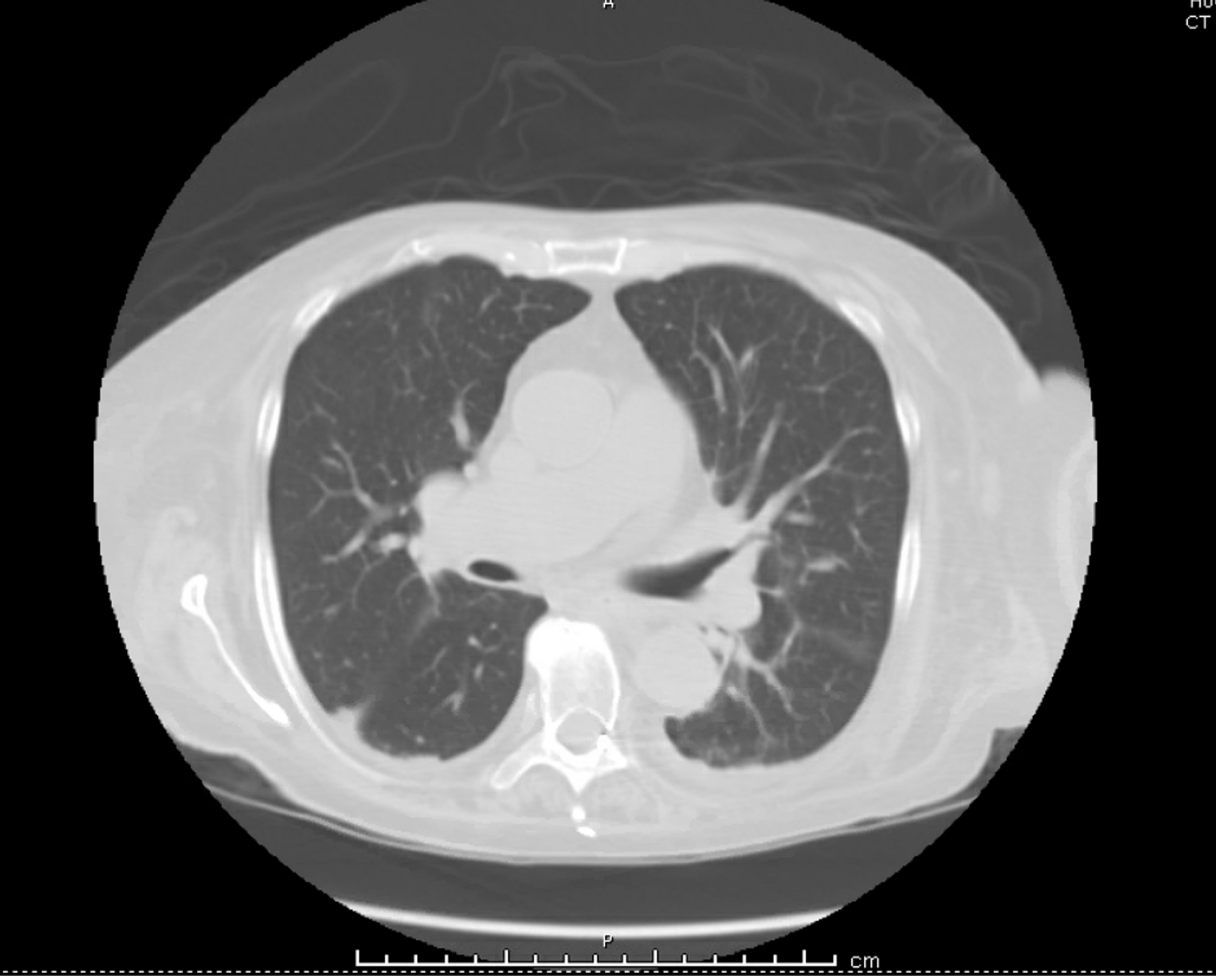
High-resolution CT of the chest reveals no evidence of parenchymal lung disease CT: computed tomography
